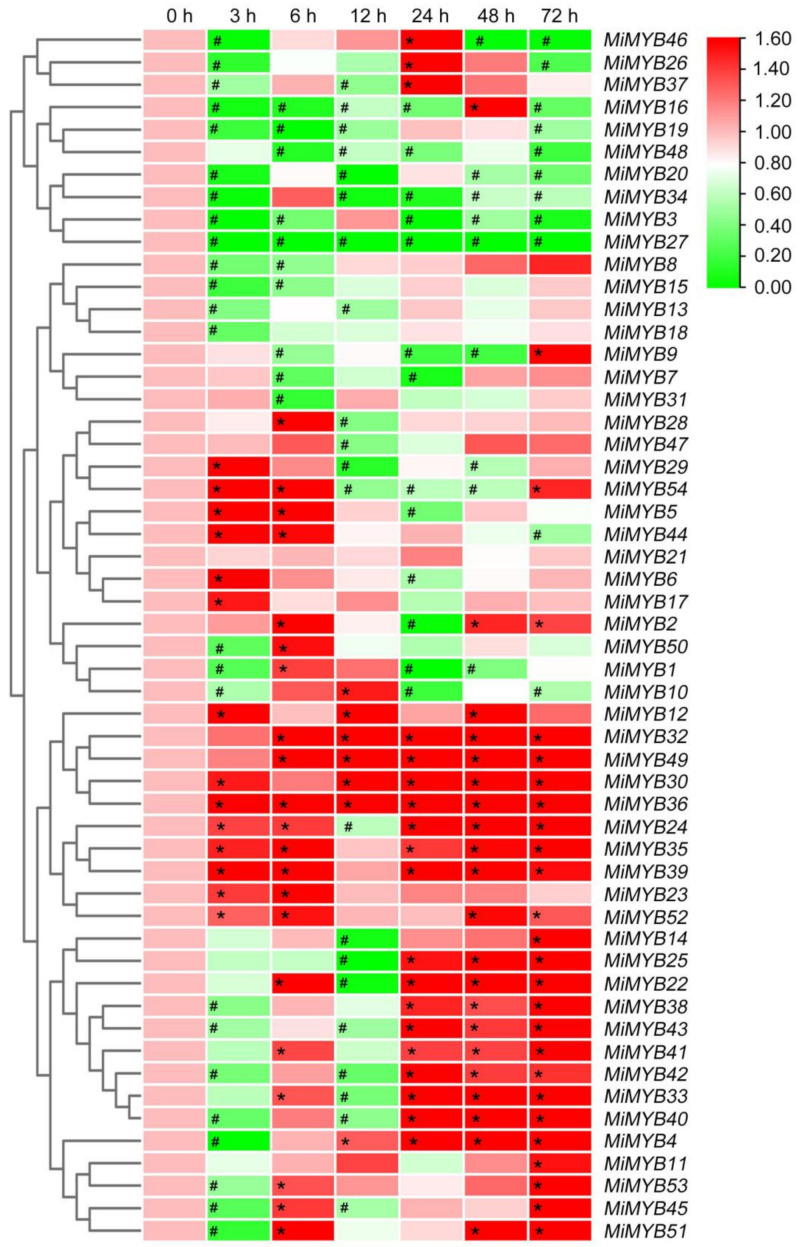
Differential expression of *MiMYBs* in response to SA treatments. Relative transcript levels of *MiMYBs* in 5 mmol/L SA treatment at 0, 3, 6, 12, 24, 48, and 72 h. The relative transcript levels of *MiMYBs* in the no-treatment mango leaves (0 h) were normalized to 1.0. The color scale represents gene expression levels with high transcript levels (red) or low transcript levels (green). The calculation method was 2^−∆∆CT^, and ‘*’ means the value exceeds 1.5, while ‘#’ means the value is below 0.5.
